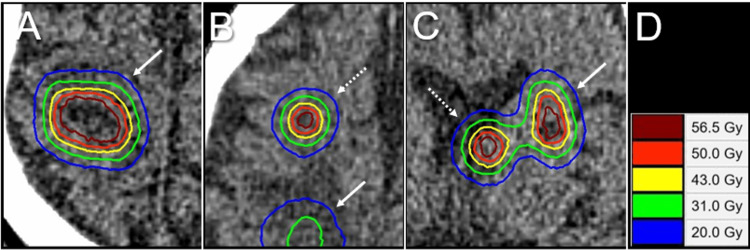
Case 1: Dose distributions for 5-fraction stereotactic radiosurgery of four brain metastases The images show (A-C) axial CT images superimposed on dose distributions for BMs in the right pre-central gyrus (arrows in A, B) (A), the right middle frontal gyrus (dashed arrow in B) (B), the left basal ganglia (arrow in C), and the left lateral ventricle (dashed arrow in C) (C); and representative isodoses (D). CT: computed tomography
